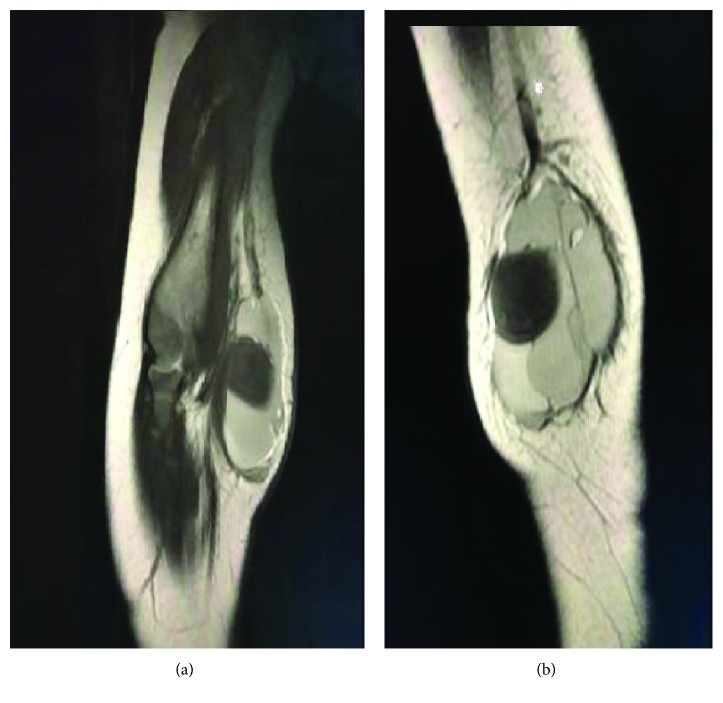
MRI of the right elbow: coronal T2-W1 sagittal T2-W1 sequences of the right elbow shows a lobular juxta articular subcutaneous soft tissue lesion along the medial aspect of the elbow; the lesion appears multiseptated with a predominantly high T2 signal intensity.
